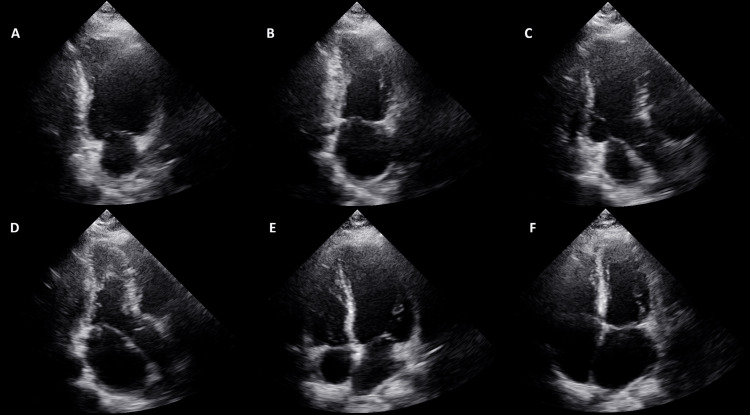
Initial transthoracic echocardiography demonstrating preserved left ventricular systolic function and no structural abnormalities. (A) Apical two-chamber view in diastole. (B) Apical two-chamber view in systole. (C) Apical three-chamber view in diastole. (D) Apical three-chamber view in systole. (E) Apical four-chamber view in diastole. (F) Apical four-chamber view in systole.
